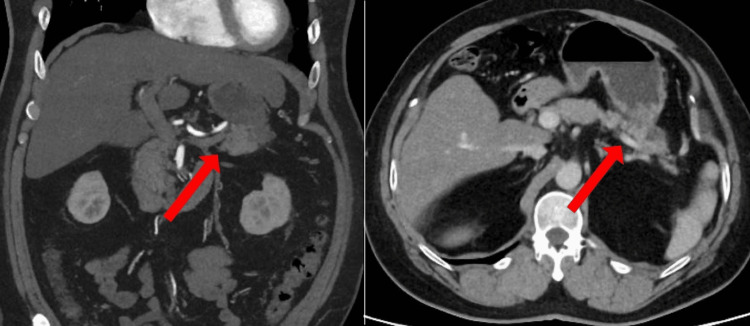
Preoperative computed tomography (CT) scan Preoperative CT scan showing a 37-mm tumor with suspected pancreatic invasion in the gastric body.
